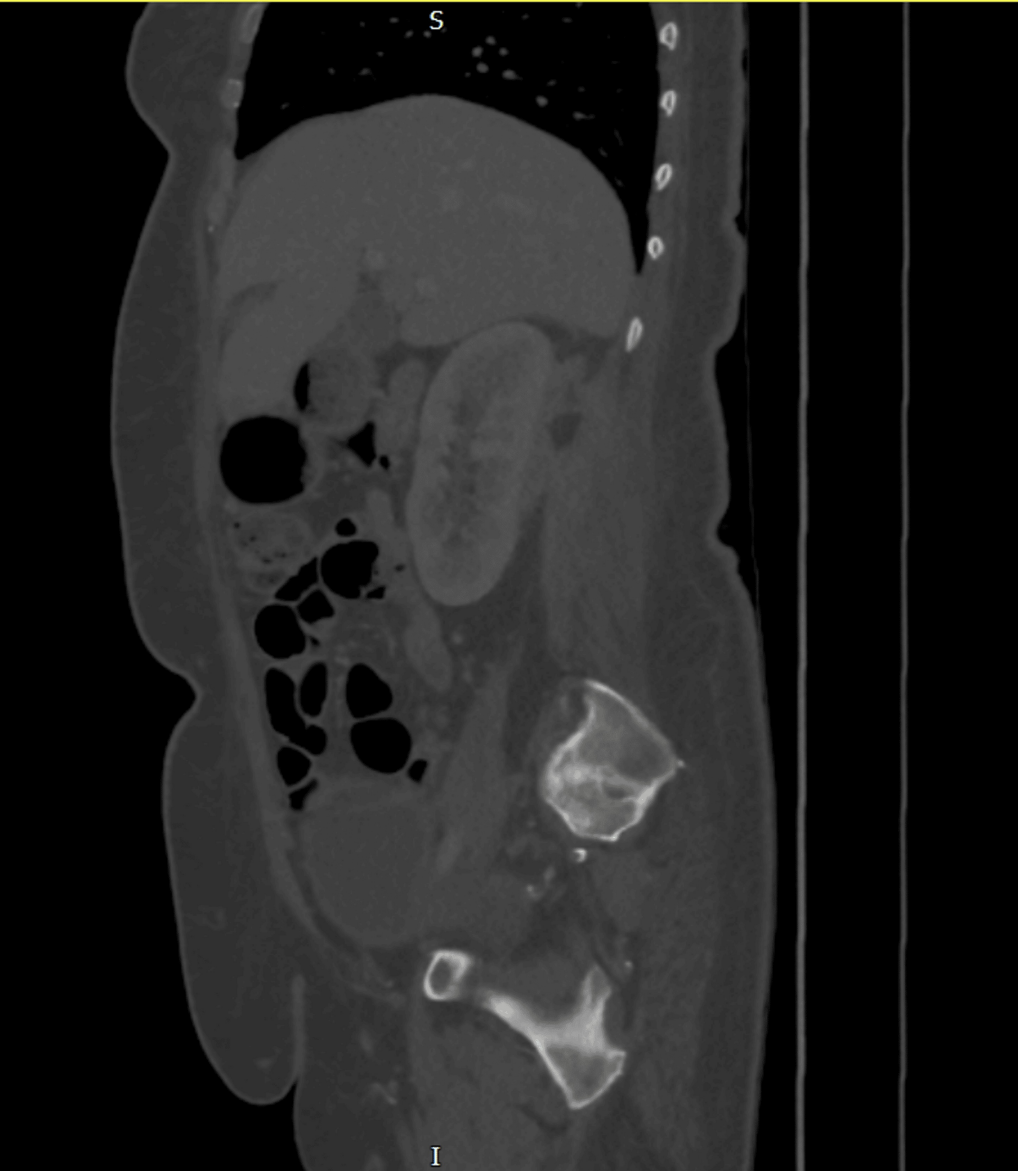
CT abdomen pelvis with contrast shows interval improvement of right perinephric collection. The collection, as before, extends into the paravertebral space but is significantly reduced. Pigtail drain is no longer in situ. The size of the abscess cannot be ascertained as it has collapsed significantly CT: computed tomography
